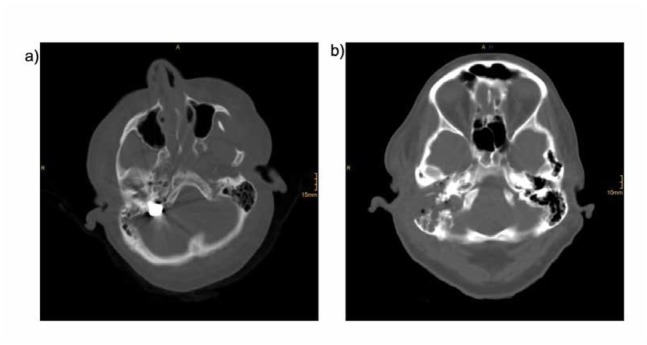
Axial computed tomography image of the base of the skull showing the bullet within the distal part of the sigmoidal sinus on the right side (a). A bony defect is seen after transmastoidal removal of the bullet (b).
